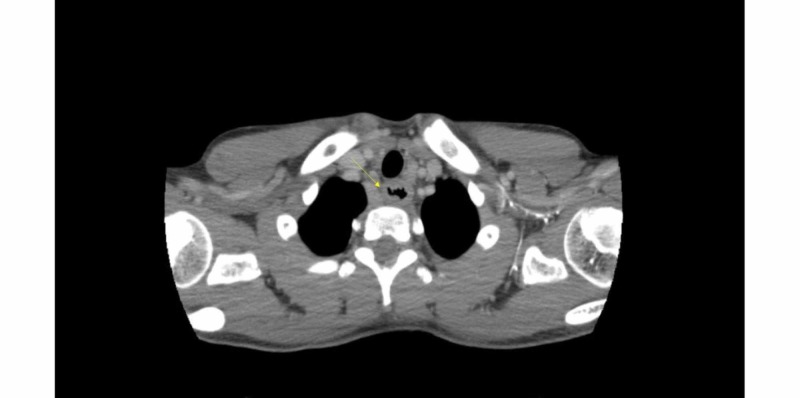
Computed tomography angiography showing circumferential thinking of the esophagus (arrow)
